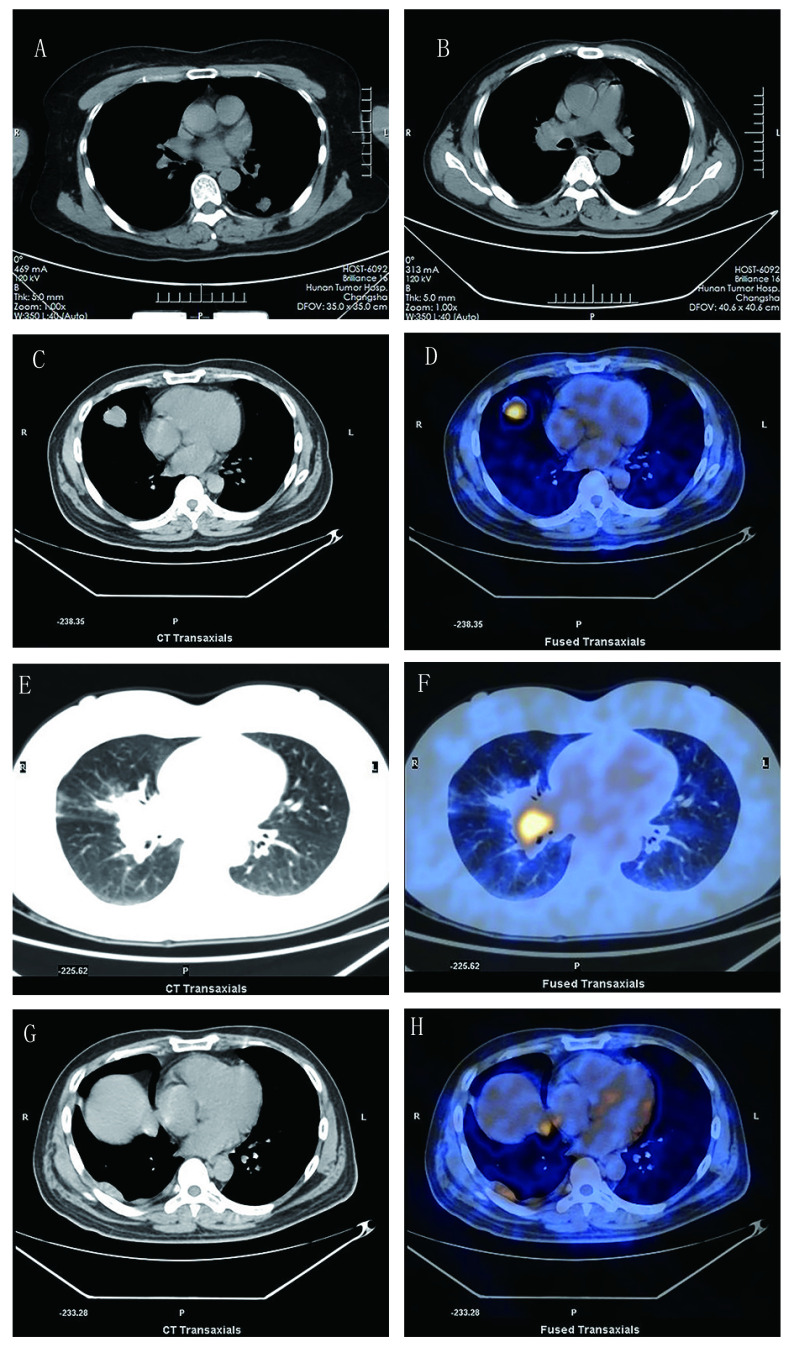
PPLELC的影像学表现。A：左肺下叶软组织结节影，分叶状，边界较清晰，密度欠均，左侧少量胸腔积液；B：化疗后CT片，右肺门区类圆形肿块影，边界不清，密度欠均；C、D：右中肺分叶状团块状影，相对应的PET-CT；E、F：右中肺近肺门区肿块影，右中肺斑片状影，相对应的PET-CT；G、H：右侧胸膜多个软组织密度结节影，相对应的PET-CT Imaging findings of PPLELC. A: A soft tissue nodule in the left lower lobe with lobular, clear boundary, inhomogeneous density and a limited amount of effusion in the left side of the chest; B: A round tumor in the right hilar area with unclear boundary and nonuniform density was demonstrated in the CT film after chemotherapy; C and D: An lobulated mass lesion in the right middle lobe of lung and the corresponding PET-CT; E and F: Mass shadow near hilum of right middle lung, patchy shadow of right middle lung and the corresponding PET-CT; G and H: Multiple soft tissue nodules were observed in the right side of the pleura and the corresponding PET-CT. PET-CT: positron emission tomography-computed tomography; PPLELC: primary pulmonary lymphoepithelioma-like carcinoma
